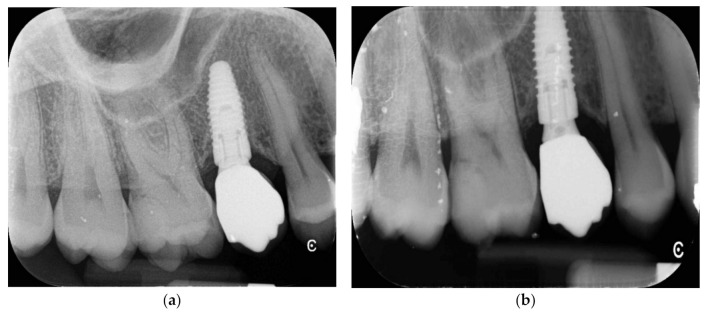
(a,b) Periapical X-rays at 5 years follow-up.
